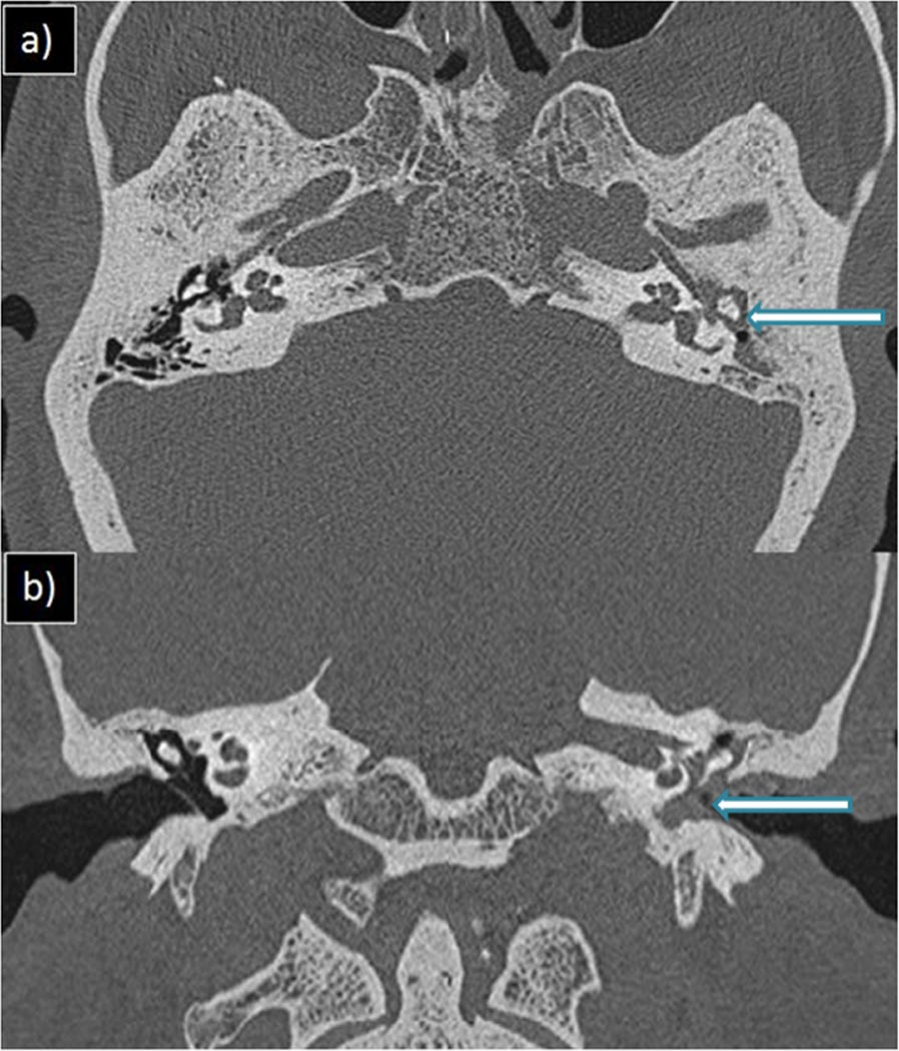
a axial and b coronal HRCT temporal bone images of 40-year-old female patient of with complaints of otalgia showing soft tissue attenuation in left middle ear and mastoid air cells with no obvious bone/ossicular erosions (arrow)—non-invasive oto-mastoiditis
